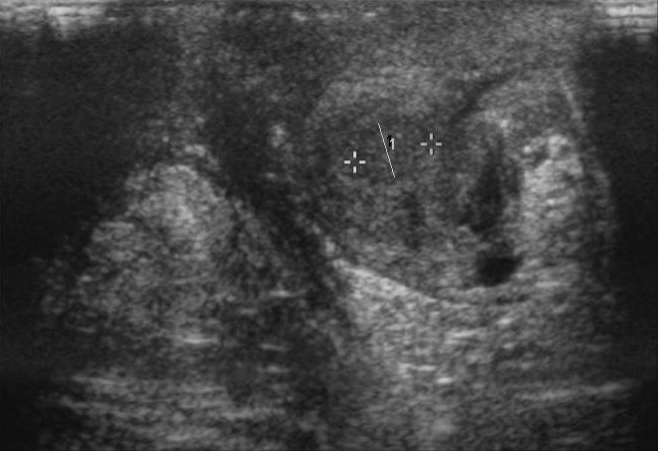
Ultrasound scan 3 months later. Ultrasound scan image showing decrease in size of the left testicle with increased nodularity in the previously injured region of the left testicle consistent with post-inflammatory scarring.
